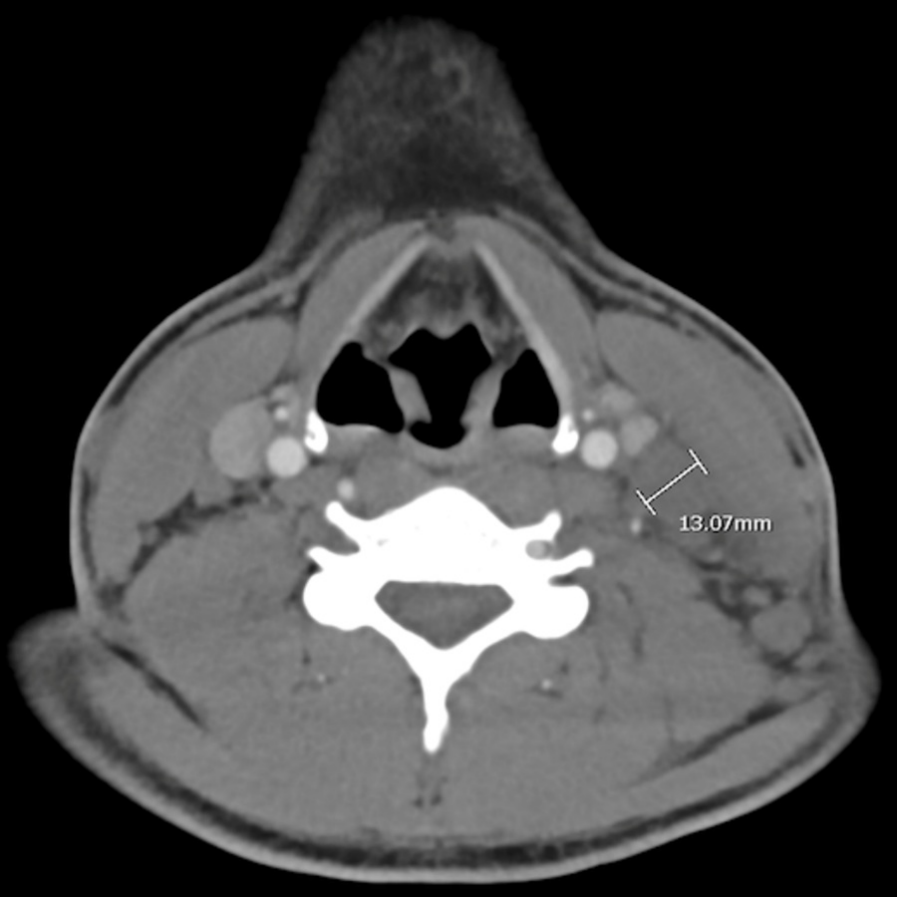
Computed tomography (CT) scan of the neck with adenopathy in the left posterior cervical carotid chain.
